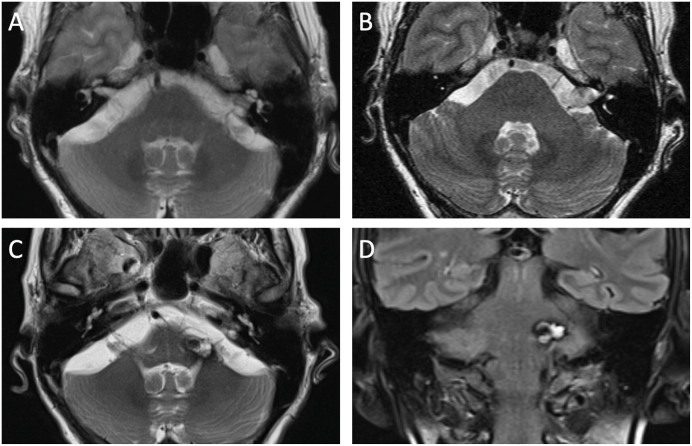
Radiation-induced cavernous malformation formation. Selection of MR images demonstrating time course of development of RICM. A) Axial T2-weigthed MR image in 2004, prior to Gamma Knife® radiosurgery (GKRS) of left vestibular schwannoma. B) Follow-up axial T2-weigthed MR image in 2017, 12 years after radiosurgery, with no evidence of a cavernous malformation. C) Axial T2-weigthed and D) coronal Fluid-Attenuated Inversion Recovery (FLAIR) MR images in 2024, 19 years after GKRS, shows a lobulated hemorrhagic lesion with mixed signal intensity and hemosiderin ring in the left cerebellar peduncle, suggestive of a cavernous malformation.
